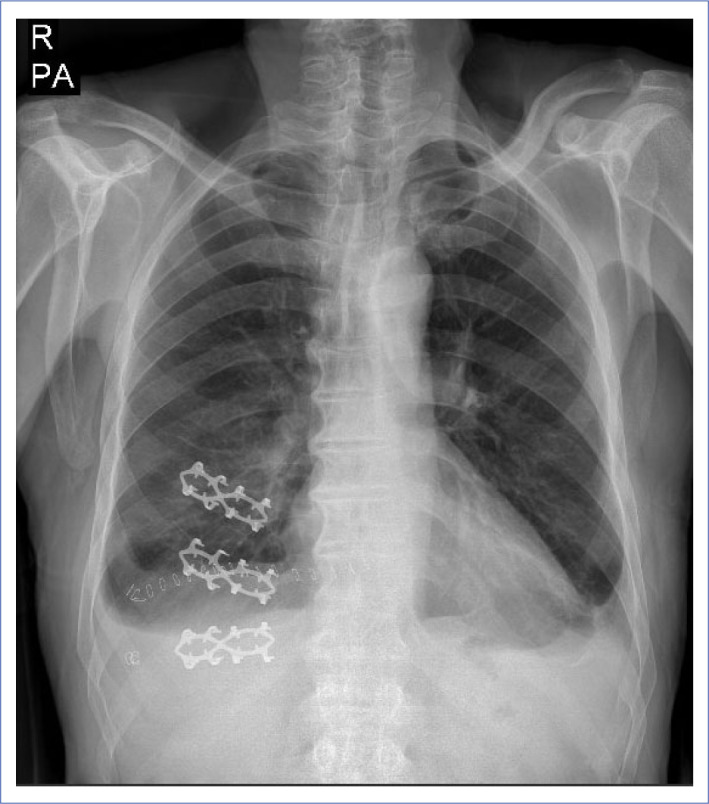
Posteroanterior chest radiograph of a post-operative case.
